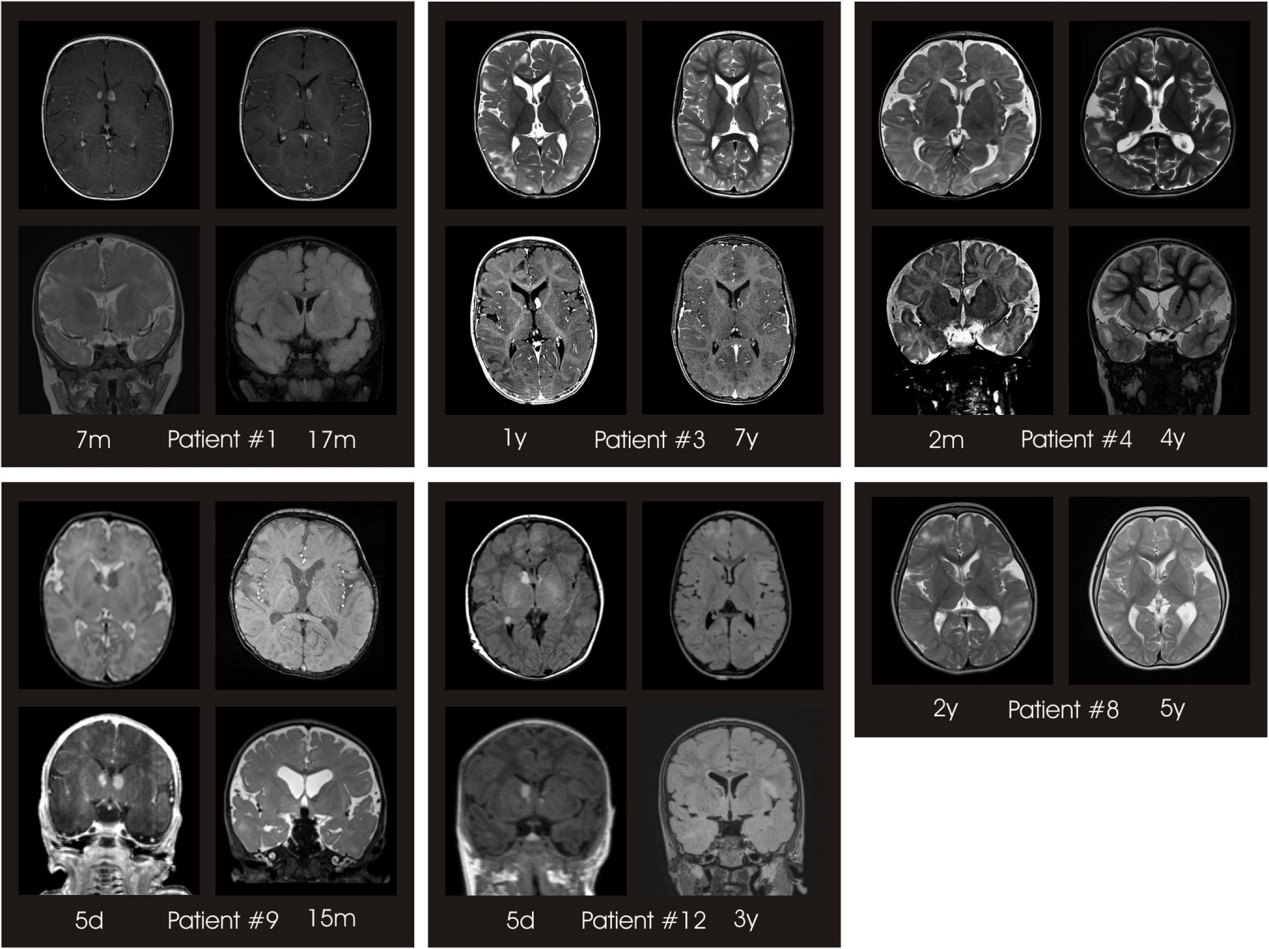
SEGA development under everolimus treatment. Axial and coronal T2-weighted and T1-weighted with gadolinium MRI sections of 6 patients treated with everolimus for SEGA. All patients were diagnosed with SEGA at risk for development of obstructive hydrocephalus at baseline *(left column)*. On follow-up in 5/6 patients marked reduction of SEGA volume was documented *(patient #1, 3, 4, 9, 12, right*
